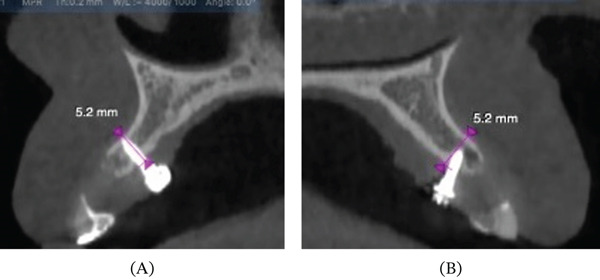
(A, B) CBCT 4 years and 6 months after placement of miniscrews.
